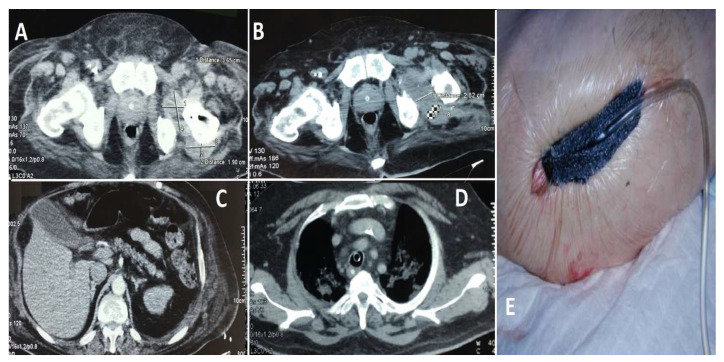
Images (A,B): The presence of a notable fluid collection in the hip region suggests the possibility of a periprosthetic infection. The presence of this accumulation was a crucial factor in the diagnosis of the infection. Image (C): A splenic lesion is observed, necessitating a splenectomy due to spontaneous rupture resulting from infectious complications. Image (D): The presence of valve vegetations on the aortic valve necessitates the performance of a valvuloplasty. The vegetations were identified through the utilization of transesophageal echocardiography, a diagnostic technique employed to assess the patient’s condition. Image (E) depicts the postoperative status of the hip, which has been fitted with a negative pressure drainage device to facilitate wound healing and reduce the accumulation of contaminated fluid.
